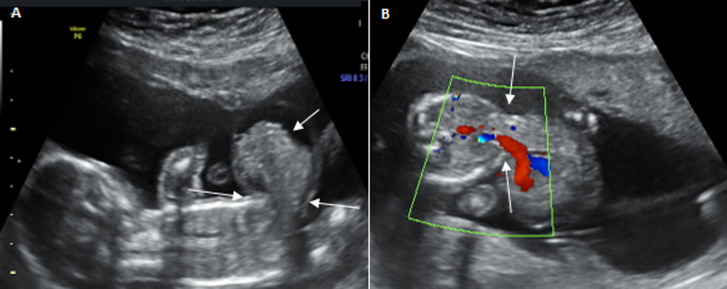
prenatal ultrasound scan images of a fetus at 20-week-GA with omphalocele, a severe congenital anomaly, in a 44-year-old woman; sagittal (A) and transverse (B) views of the fetus showing a defect in the anterior abdominal wall (arrows) with extrusion of the abdominal content through the defect and a membrane is seen to cover the abdominal content; the umbilical vein is seen centrally within the extruded abdominal contents (red colour)
